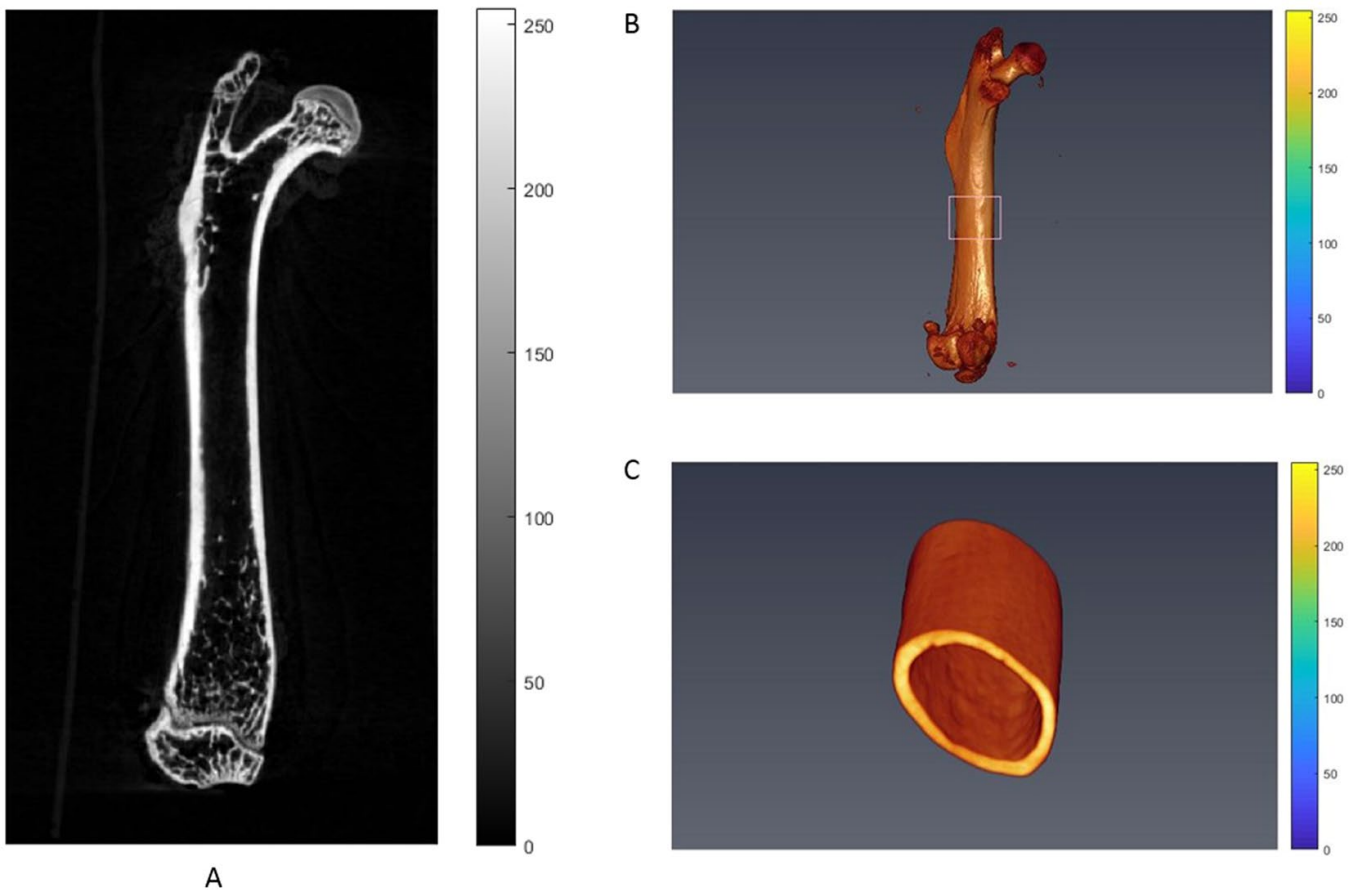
(A) An image of a coronal view of a femur bone acquired from the micro CT scanning. The region of interest is shown in the added box. (B) A 3D volume-rendered image of a femur bone acquired from the micro CT scanning. The region of interest is shown in the added box. (C) A 3D volume-rendered image of the region of interest.
